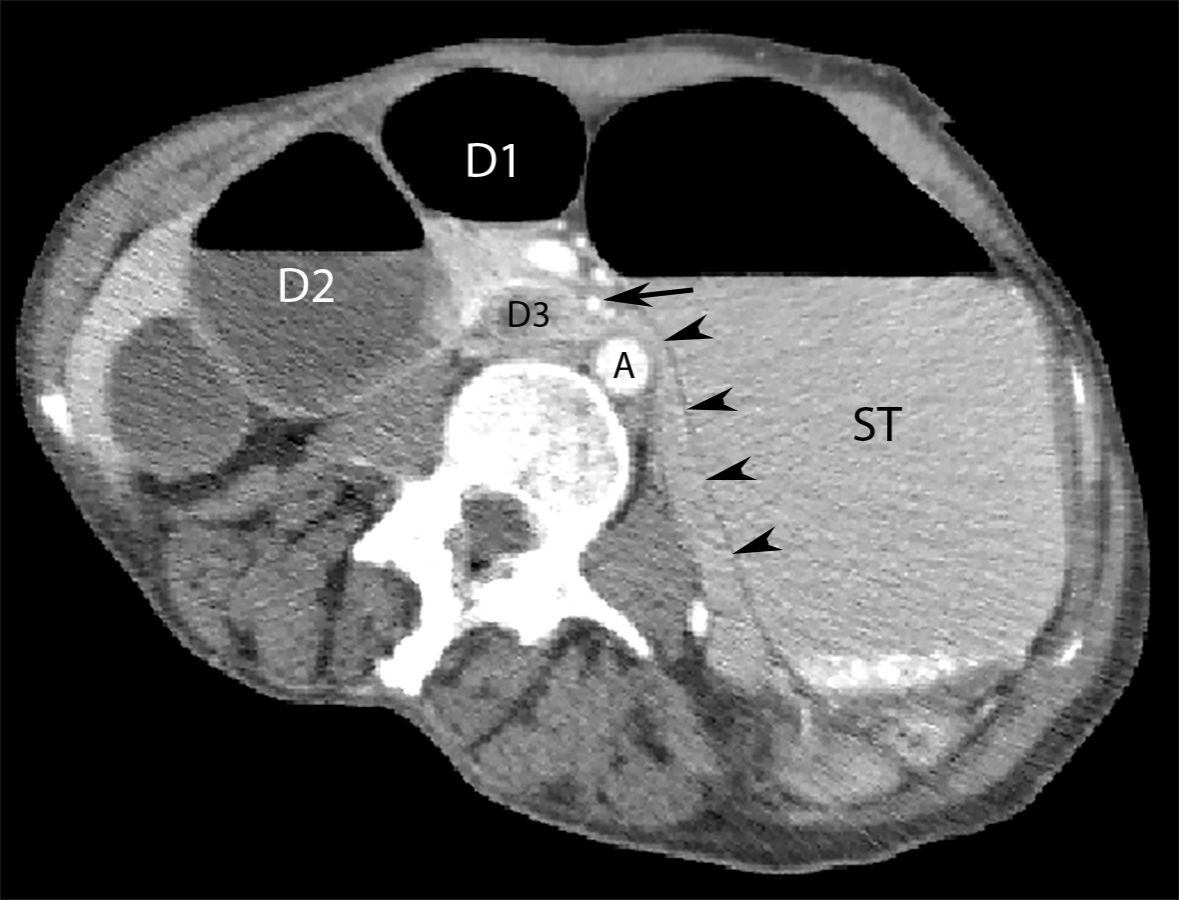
Computed tomography (CT) scan (axial view) of abdomen raising suspicion of superior mesenteric artery (SMA) syndrome. The figure depicts a computed tomography (CT) scan of abdomen showing dilatation of stomach (ST) and the first (D1) and second (D2) part of duodenum with abrupt collapse of the third part of duodenum (D3) at the point where it crossed posterior to SMA (arrow). Arrowheads also represent the collapsed duodenum.
